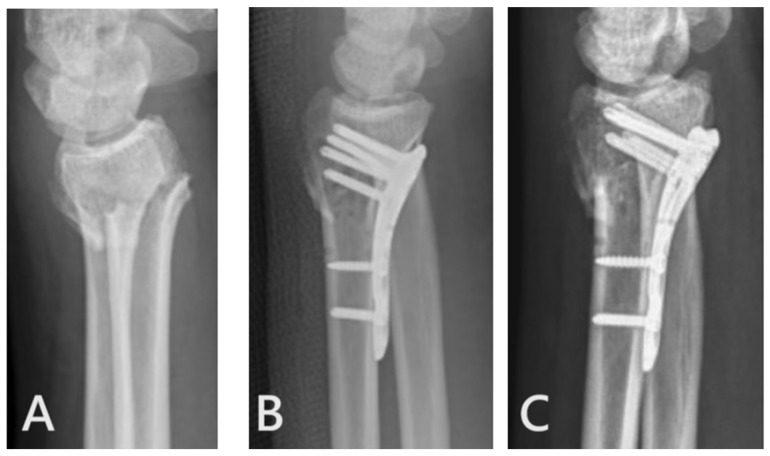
Re-displacement of fracture. (A) Pre-operative X-ray. (B) Immediate post-operative X-ray. (C) Follow-up X-ray.
